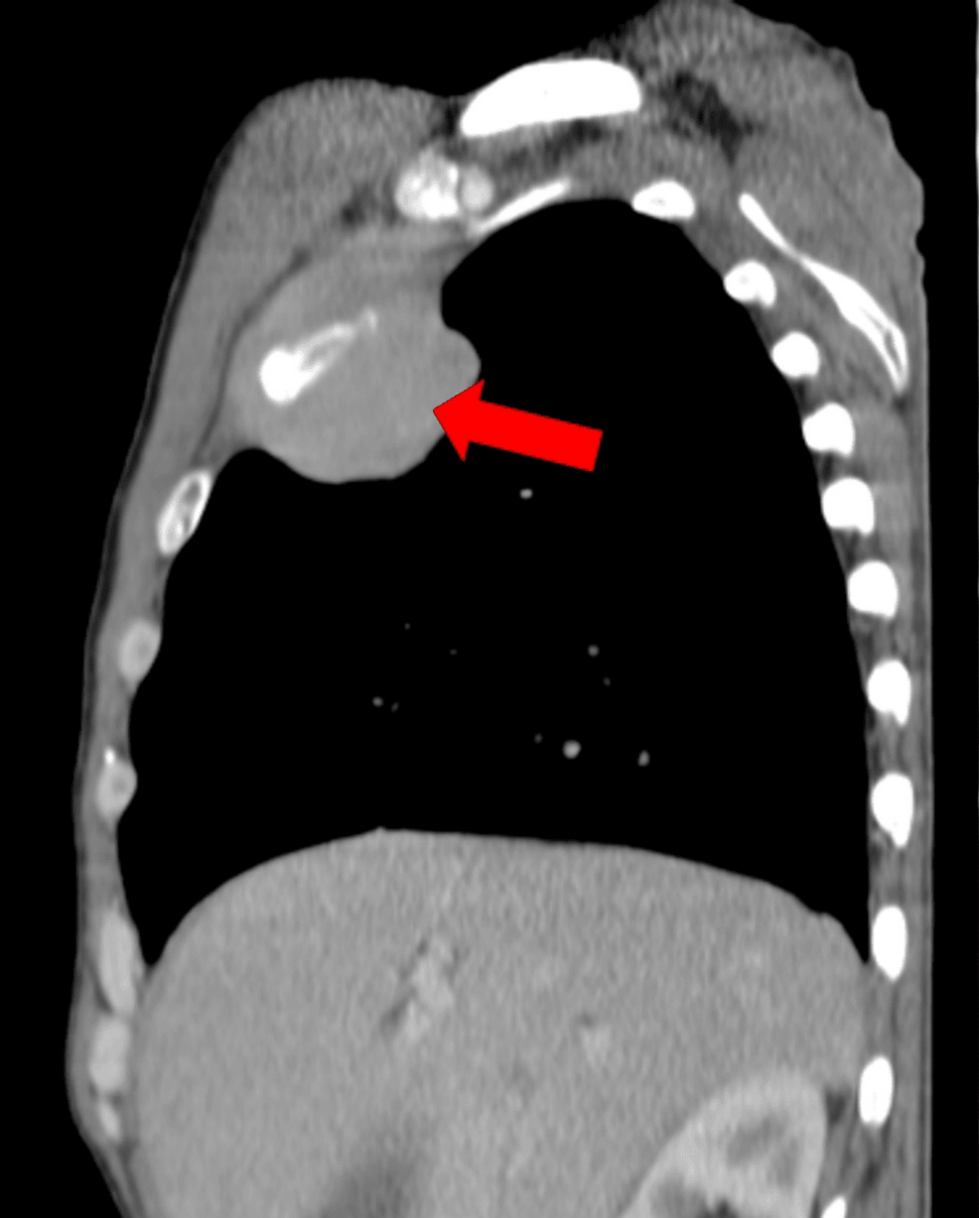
Thoracic CT scan. A 2.7 cm solid mass just to the right of the sternum.
